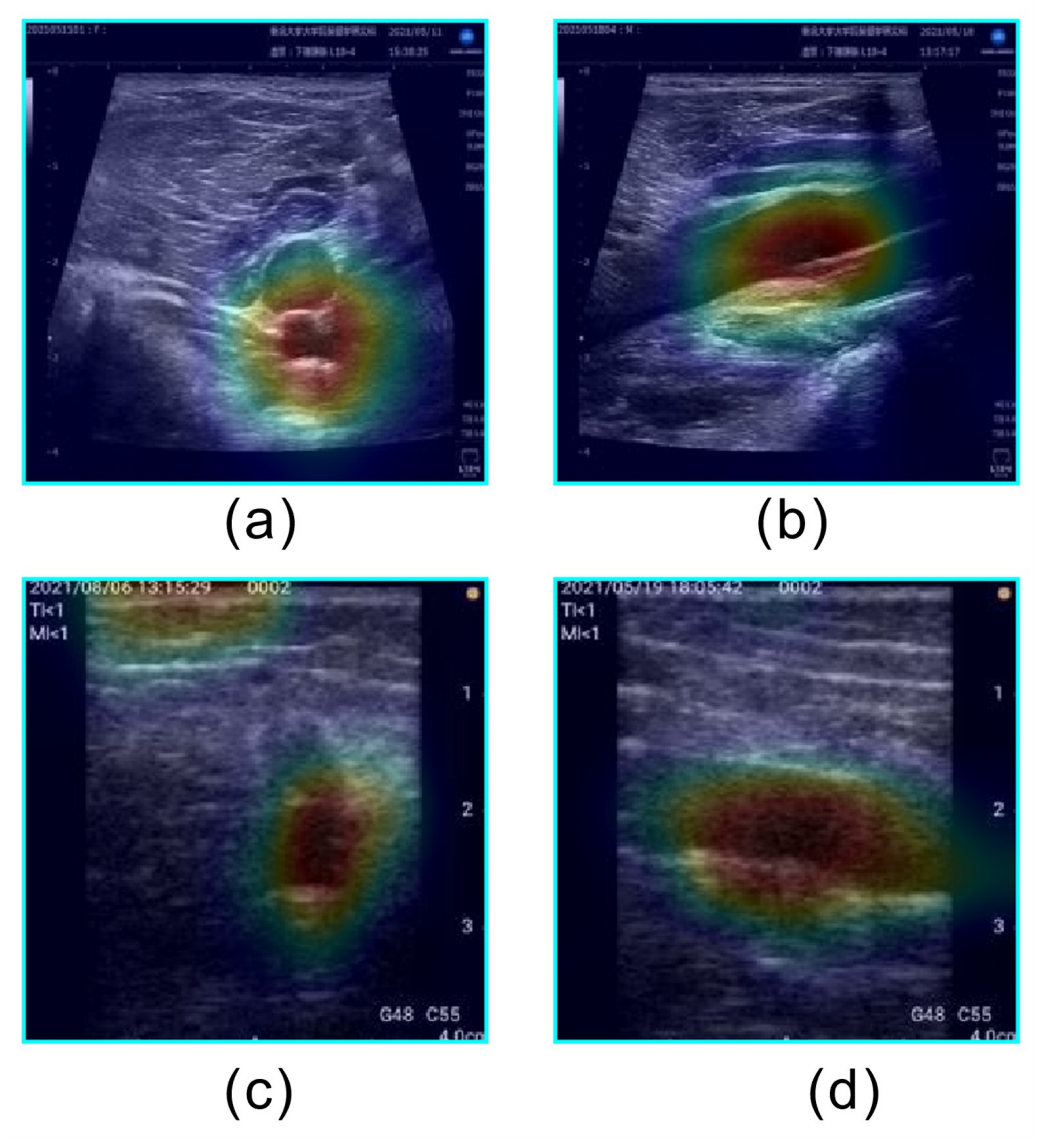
Examples of heatmaps for correctly classified satisfactory images. (a) Short axis acquired by stationary ultrasonography equipment, (b) Long axis acquired by stationary ultrasonography equipment, (c) Short axis acquired by portable ultrasonography equipment, and (d) Long axis acquired by portable ultrasonography equipment.
